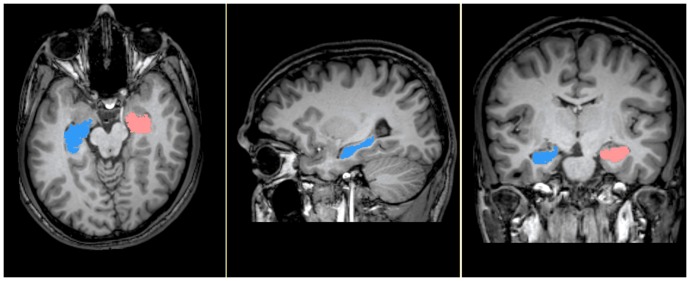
Cerebral MRI depicting manual segmentation of hippocampus. Figure shows MRI with segmented hippocampus in axial, sagittal & coronal sections within 3D-Slicer software interface.
